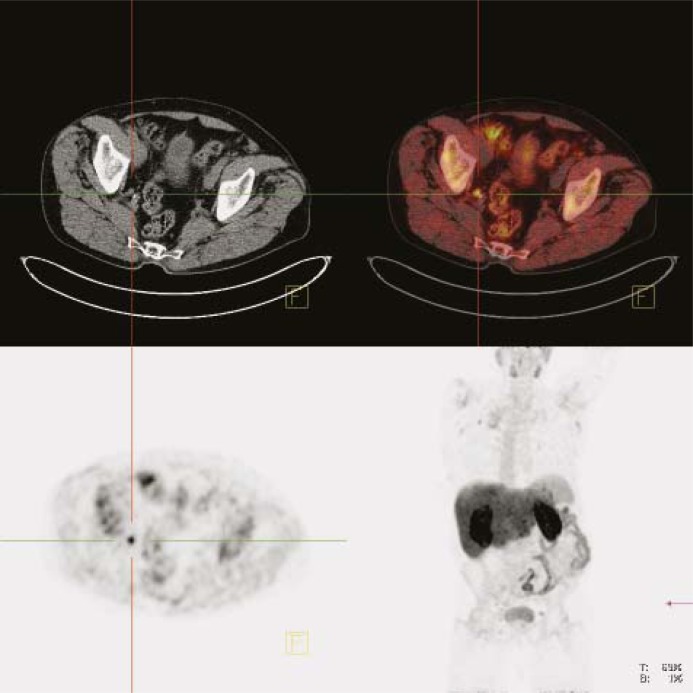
Lymph node metastases due to prostate cancer: whole body scan (late images). Upper left panel: CT image. Upper right panel: fused PET/CT image. Lower left panel: PET image. Lower right panel: maximum intensity projection (MIP).
